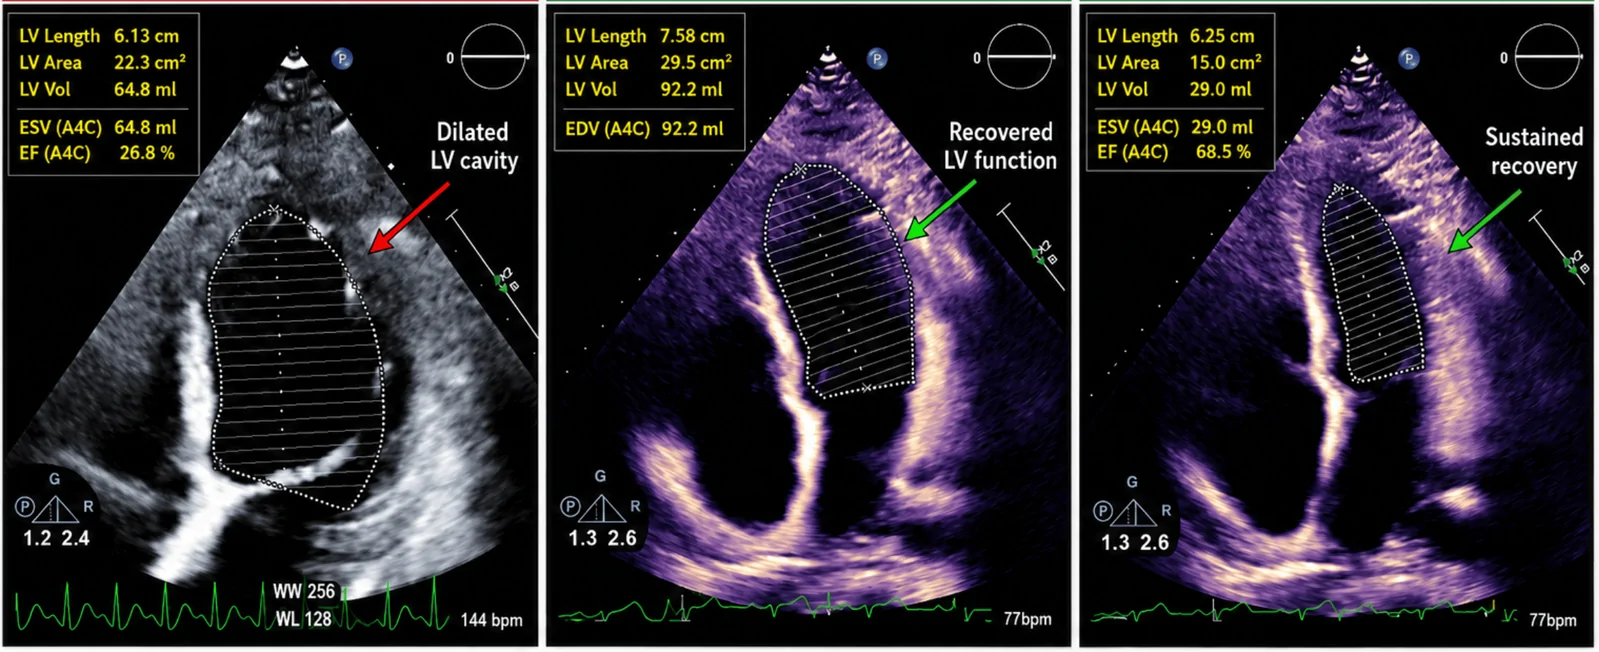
Transthoracic echocardiography demonstrating recovery of left ventricular systolic function. Apical four-chamber transthoracic echocardiographic views with left ventricular tracing. The initial study demonstrated markedly reduced left ventricular systolic function in the setting of acute amphetamine-associated cardiomyopathy, while follow-up imaging showed normalization of left ventricular ejection fraction to 68.5%, supporting reversible stress/toxin-mediated cardiomyopathy.
